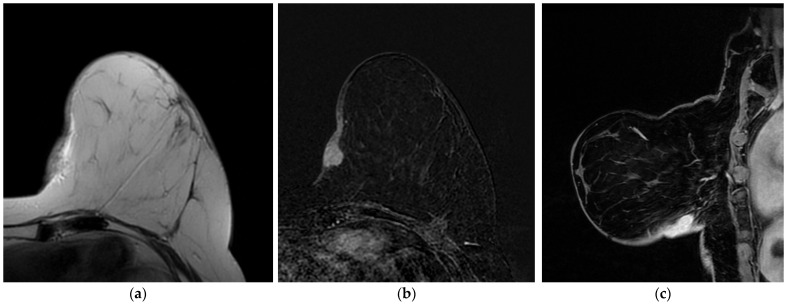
A 48-year-old patient with a history of left breast cancer treated with quadrantectomy and radiotherapy. (a) T2-weighted, (b) axial 3D gradient echo T1-weighted post-contrast and (c) sagittal 3D gradient echo T1-weighted post-contrast images show skin thickening and an oval-shaped mass, with circumscribed margins, characterized by homogenous enhancement. US-guided core needle biopsy reveals SBA.
